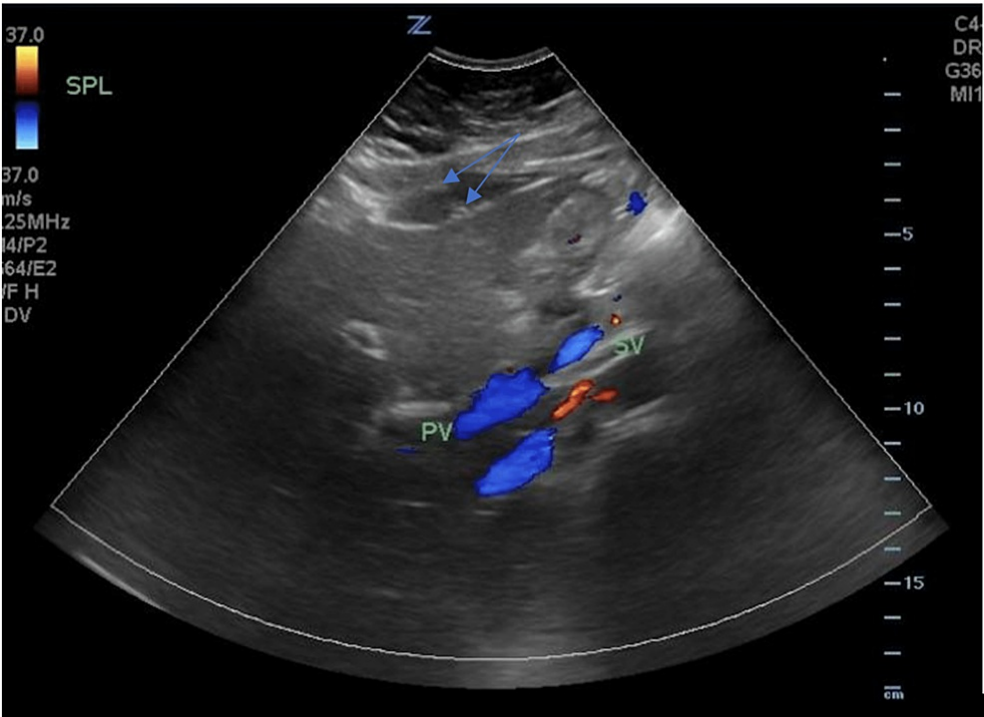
The ultrasound of the right upper quadrant of the abdomen shows multiple stones along the gallbladder without associated gallbladder wall thickening or pericholecystic fluid.
